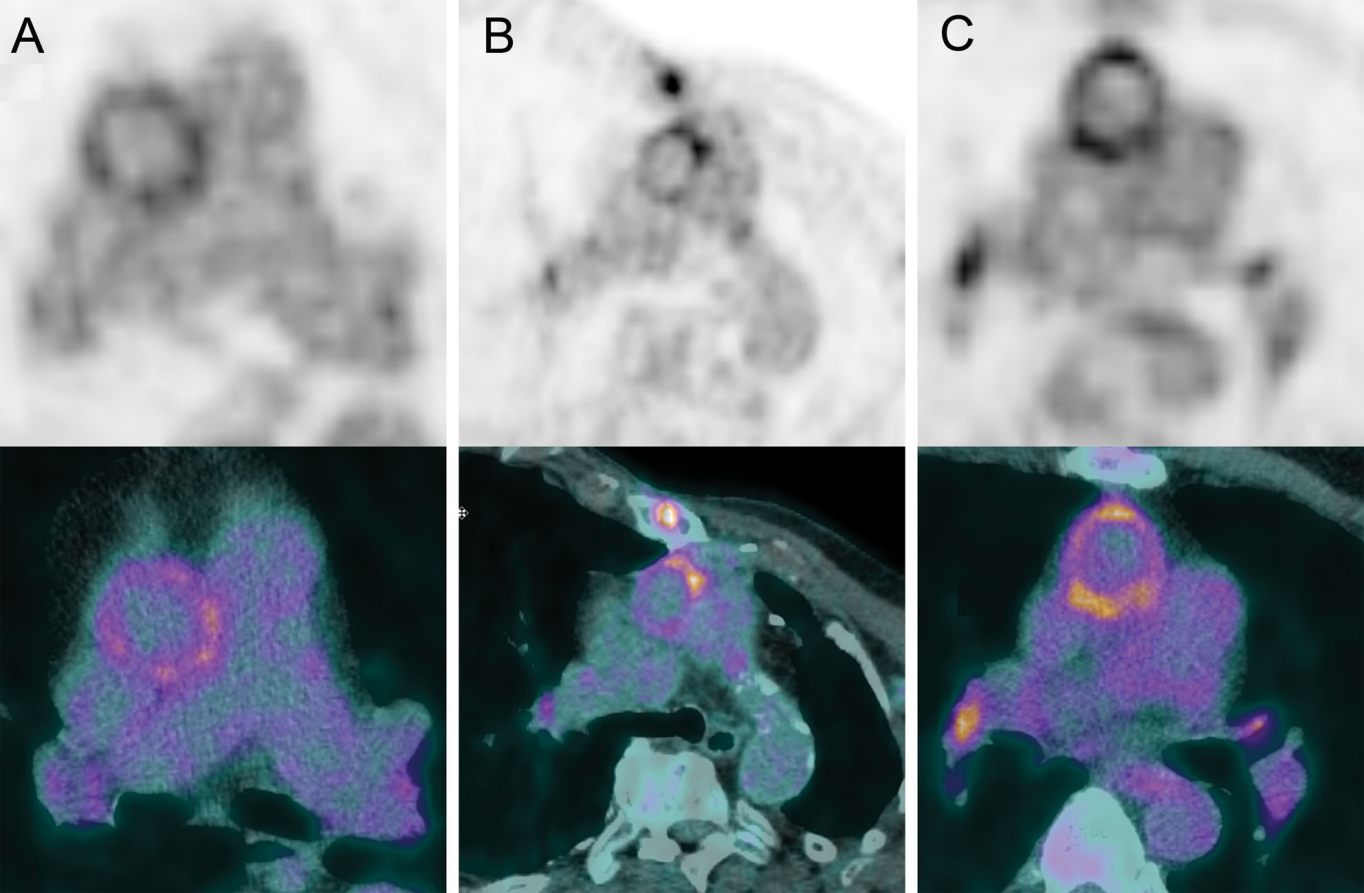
Examples of ^18^F-FDG uptake patterns (A: diffuse homogeneous, B: focal, C: diffuse heterogeneous) around the cranial anastomosis. Scaling was set the same for all images and represents SUV with a range of 0-7
